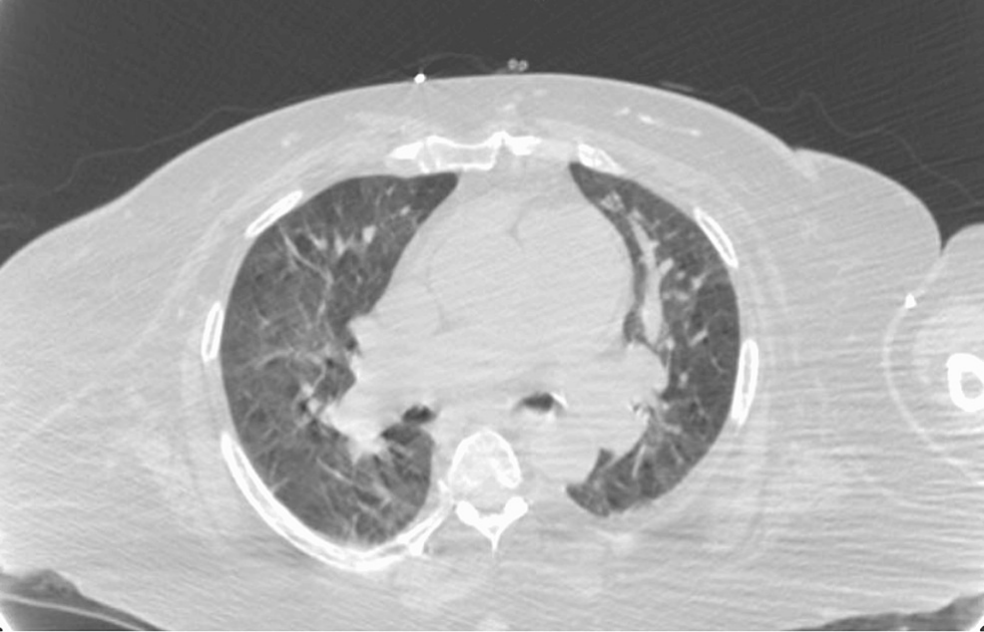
Computed tomography of the chest with evidence of bilateral opacities and pulmonary edema consistent with volume overload.
